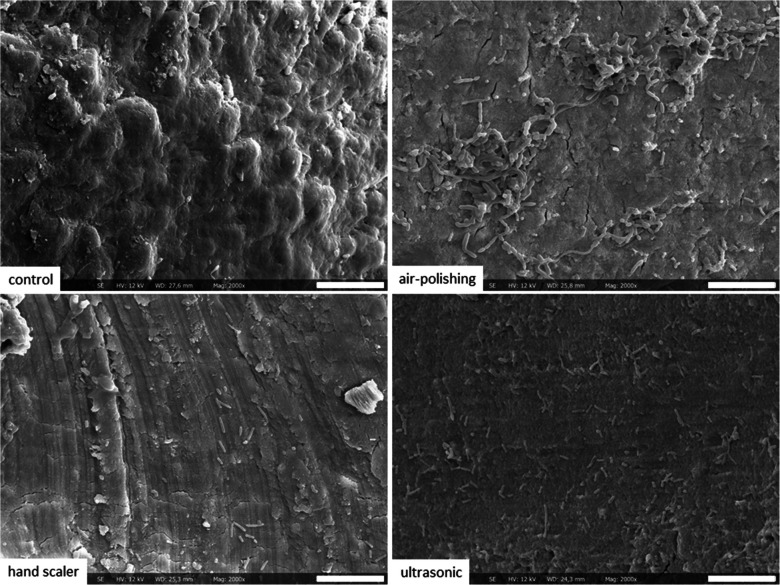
SEM photographs of the root surfaces below the cementum-enamel junction without treatment (top left) and after receiving instrumentation with a manual scaler (down left), with air polishing (top right) or with an ultrasonic scaler (down right) and subsequent cultivation with a 6-species mixture for 24 h (400-fold magnification, bar 60 μm)
